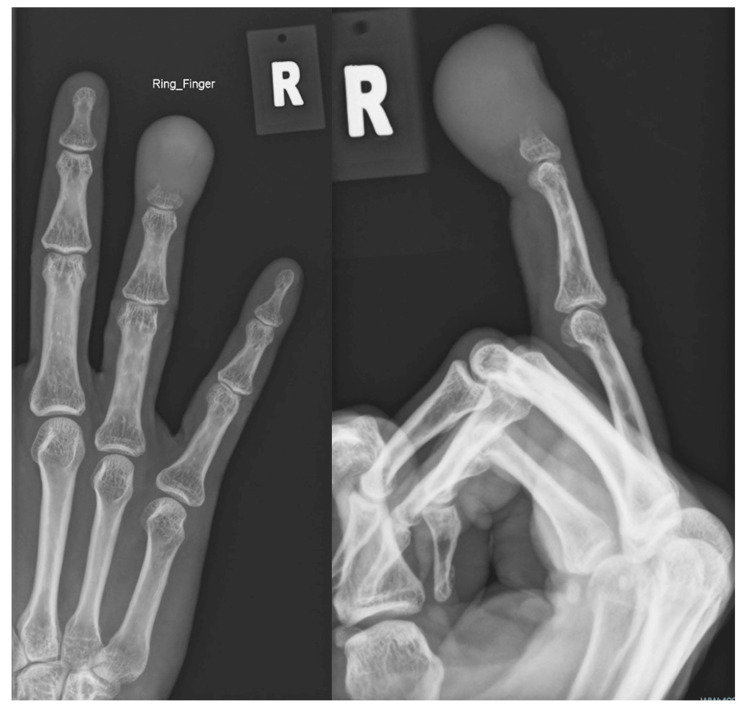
X-ray of patient's right ring finger in A/P (left) and lateral (right) views Both images demonstrate a soft tissue mass in the pulp of the distal phalanx of the right ring finger, with erosion of underlying bone.
